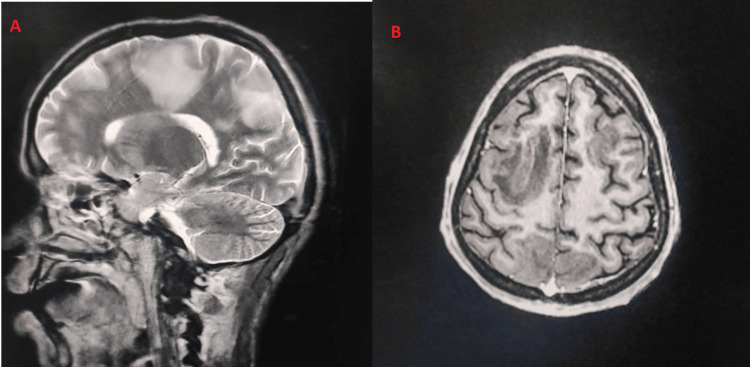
MRA images in a case of CNS vasculitis A) T2-weighted sagittal image of the brain showing patchy areas of hyperintensities involving the right frontal and parietal lobes with predominant involvement of subcortical and deep white matter. Features are consistent with the area of demyelination. B) T1-weighted axial image of the brain shows sharply defined patchy hypointense areas involving the bilateral frontal lobes and parietal lobes. MRA: Magnetic resonance angiography.
